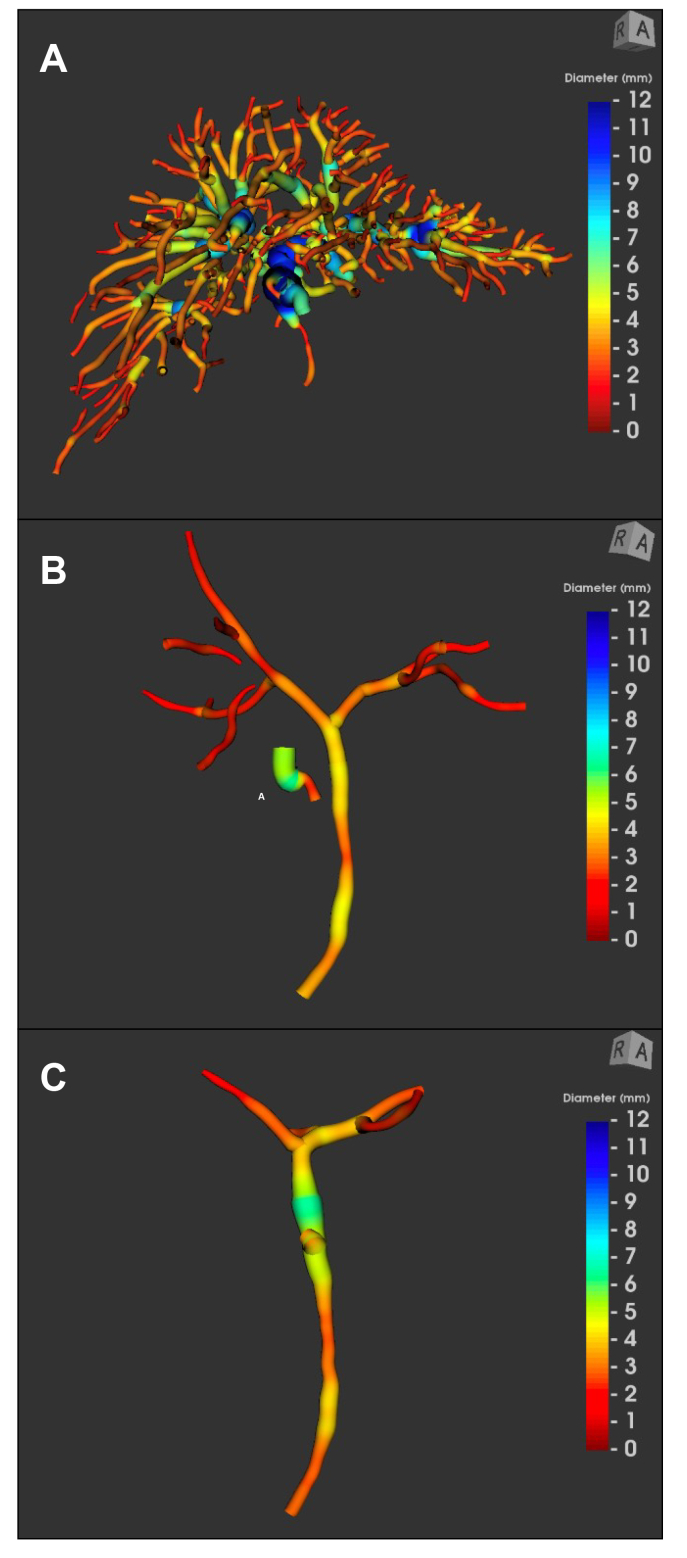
Visual difference between MRCP+ with similar proportion of ducts with median 3–5-mm diameter. (A) Visualises the MRCP+ acquisition of a large-duct PSC patient with a 34% (83/244 ducts with median 3–5-mm diameter) proportion of median 3–5-mm diameter ducts while (B) shows a small-duct patient with a 37% (10/27 ducts) proportion and (C) a healthy control with a 37.5% (three of eight ducts) proportion of 3–5-mm diameter ducts. This figure emphasises the increased load of intrahepatic ducts that have crossed the 1 mm MRI resolution threshold in patients with PSC. MRCP, magnetic resonance cholangiopancreatography; MRI, magnetic resonance imaging; PSC, primary sclerosing cholangitis.
